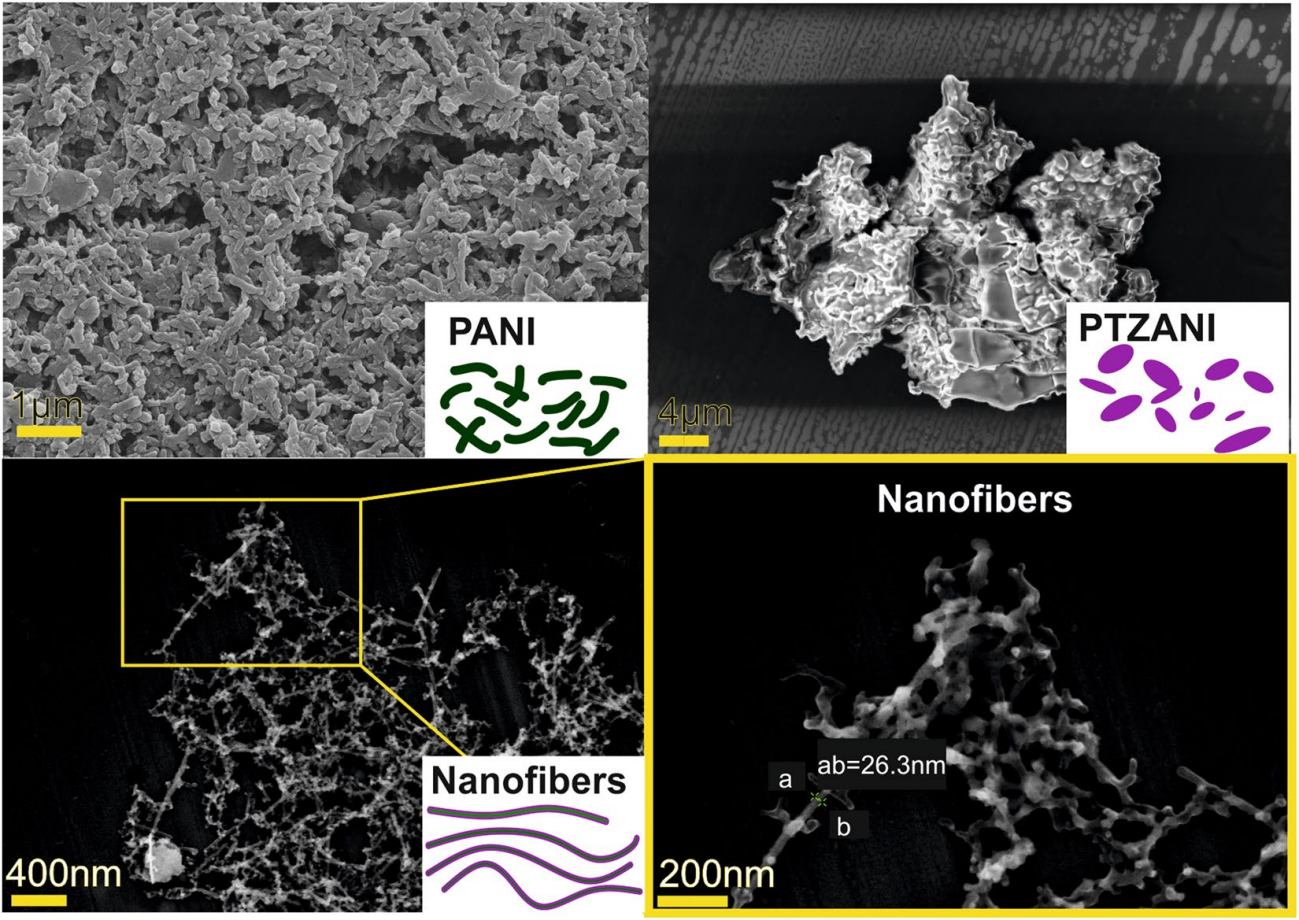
SEM images of (a) emeraldine (b) mixture of emeraldine and PTZANI obtained by stirring (c,d) nanofibers of emeraldine and PTZANI obtained by ultrasonication
